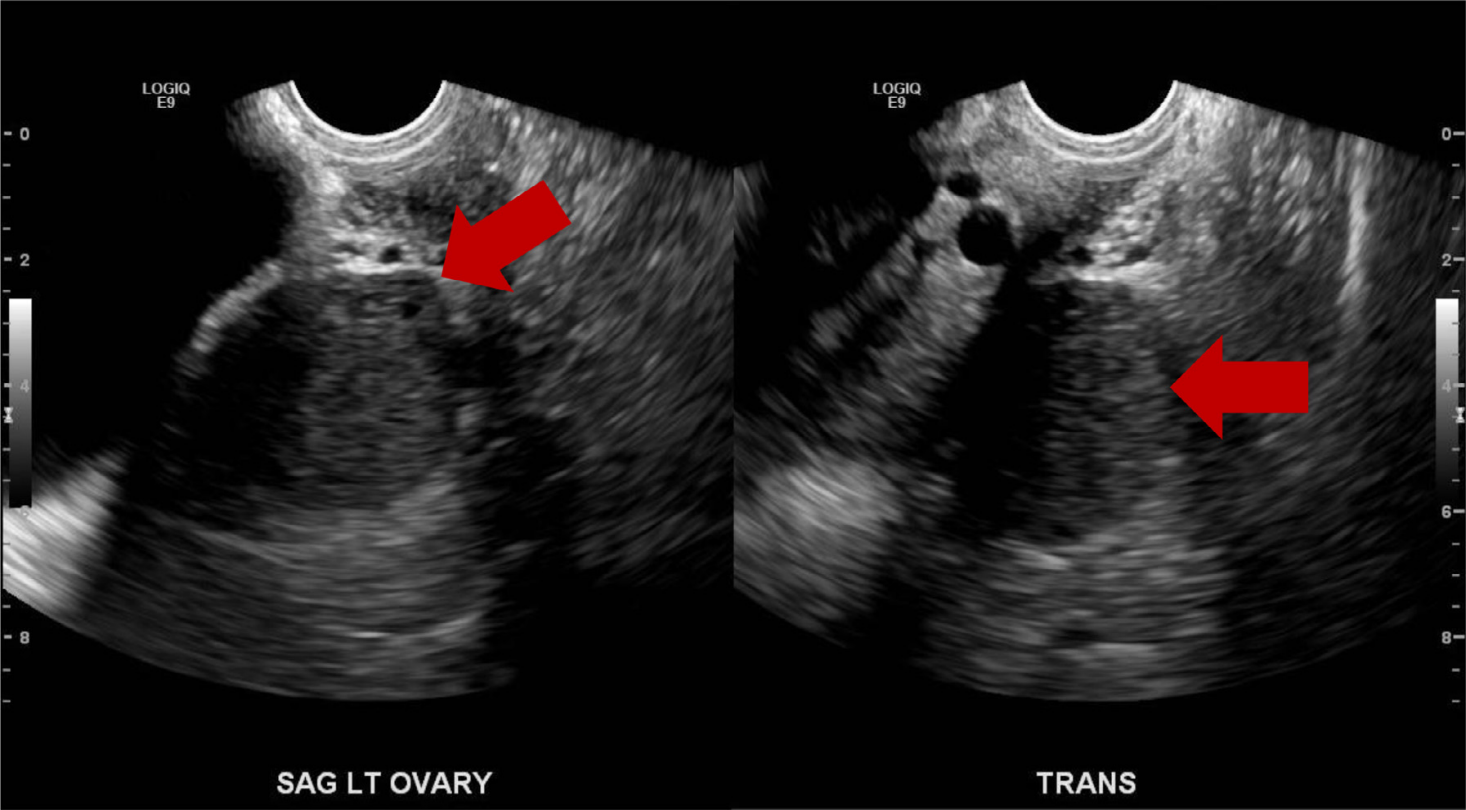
(A and B) Transvaginal ultrasound of left ovaria (in grayscale). Both sagittal and transverse images demonstrate a hypoechoic, solid mass in the left ovary.
